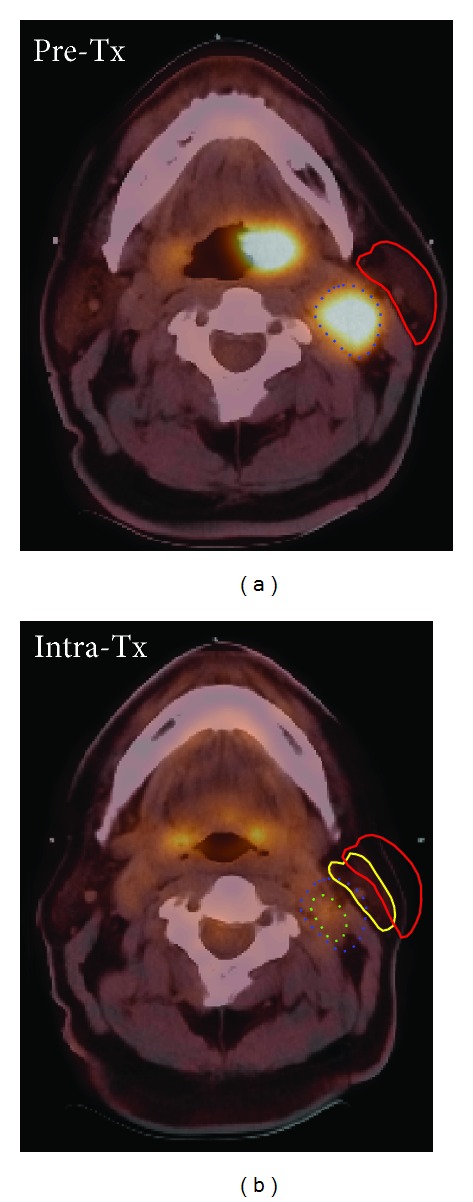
Both tumors and normal tissues may shrink and shift during the treatment. The coregistered PET/CT images of Pre-Tx (a) and Intra-Tx (b) are cross-sectional images of a patient with a primary tumour of the tonsil. The patient's left parotid gland in Intra-Tx (yellow contour) shows volume loss and shift relative to that in Pre-Tx (red contour). Similarly, the gross tumour volume for one nodal disease site in Intra-Tx (dotted green contour) shows volume loss and shift relative to that in Pre-Tx tumour (dotted blue contour).
